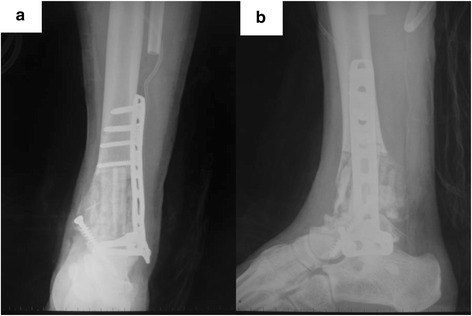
Post-operative X-ray shows the reconstruction of the distal tibia defect. a AP X-ray. b Lateral X-ray
